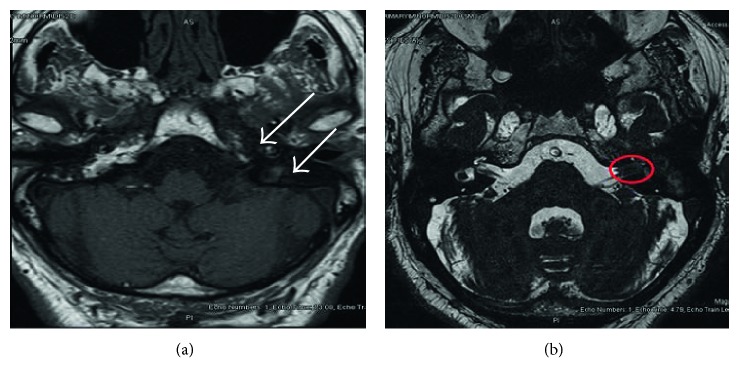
(a) MRI with contrast showing asymmetrical signal changes in the bilateral petrous bone with reduced enhancement on the left with high suspicion of petrositis. (b) Contrast showing an enhancing lesion in the internal auditory meatus involving the 7th-8th nerve complex most likely acoustic neuroma (red circle).
